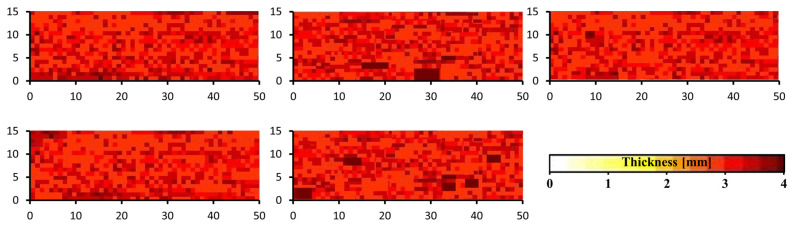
Mapped scans of the windows investigated by ultrasonic inspection on 15 × 50 mm wide scanning windows.
